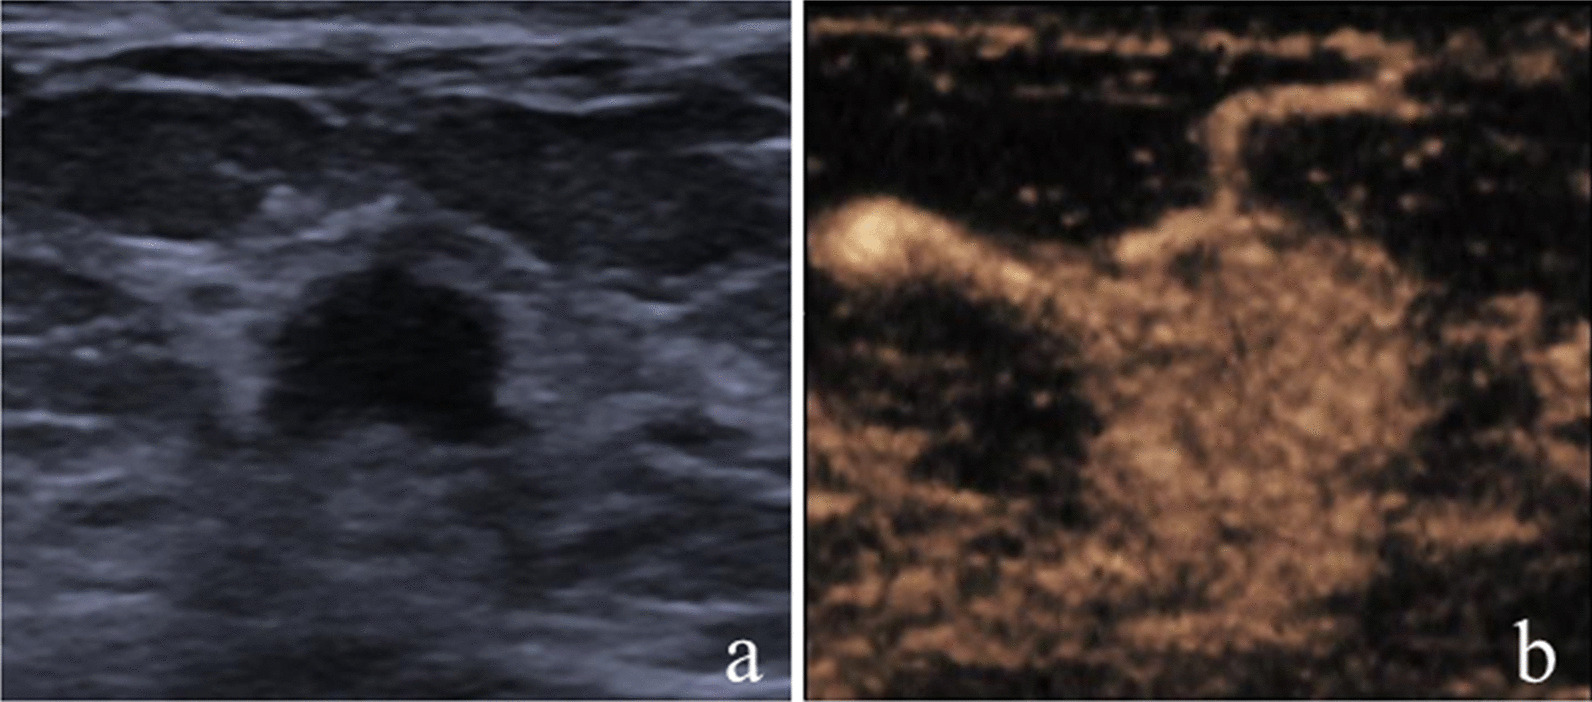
Image of a 42-year-old woman with a nonpalpable mass. a B-mode ultrasound image; b contrast-enhanced ultrasound image showed that the lesion displayed hyper-enhancement with ill-defined enhancement margin, enlarged size on CEUS, and penetrating vessels. The CEUS score was 5 points. This lesion was originally classified as US BI-RADS 4A, upgraded to BI-RADS 4B after CEUS, and diagnosed as atypical ductal hyperplasia via US-guided core needle biopsy and as ductal carcinoma in situ at surgery
